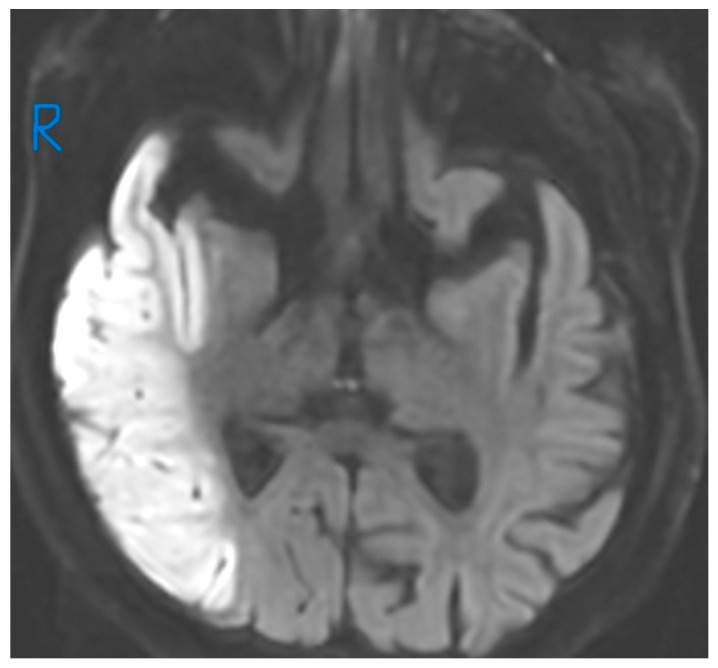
Neuroimaging findings taken 3 h after the onset of clinical signs. Acute infarct and its volume on DWI sequence—129,785 cm^3^. (“R” for right side of the image).
